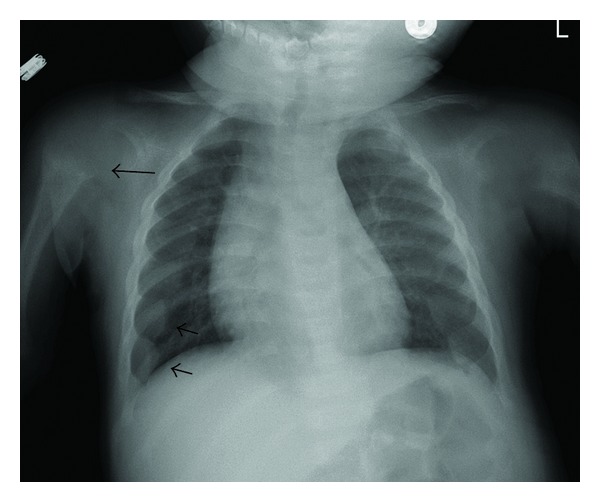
Chest radiograph of an 8-month-old male with fever demonstrating widened anterior ribs (short arrows) and proximal humeral metaphyseal fraying and cupping (long arrows). (Reprinted with permission of the American College of Radiology (ACR's Case in Point, March 20, 2012, Rickets). No other representation of this material is authorized without expressed, written permission from the American College of Radiology).
